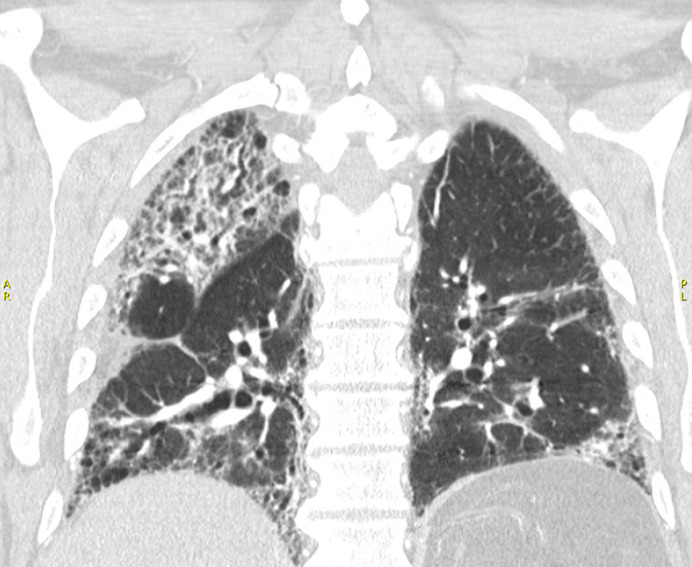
coronal CT lung window demonstrating mixed reticulation, fibrosis and honey combing; note the relative sparing of the left upper lobe
